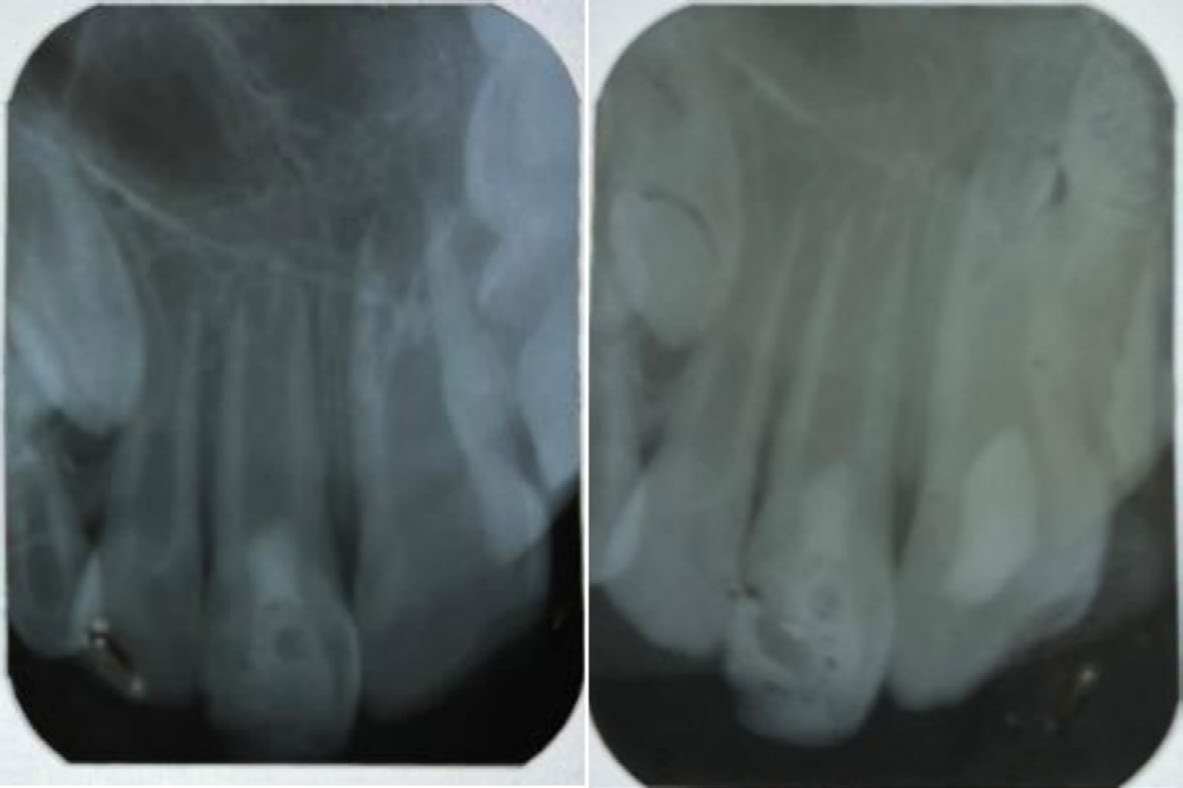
Radiographs of cervical pulpotomy procedures (1 month after initial visit).
